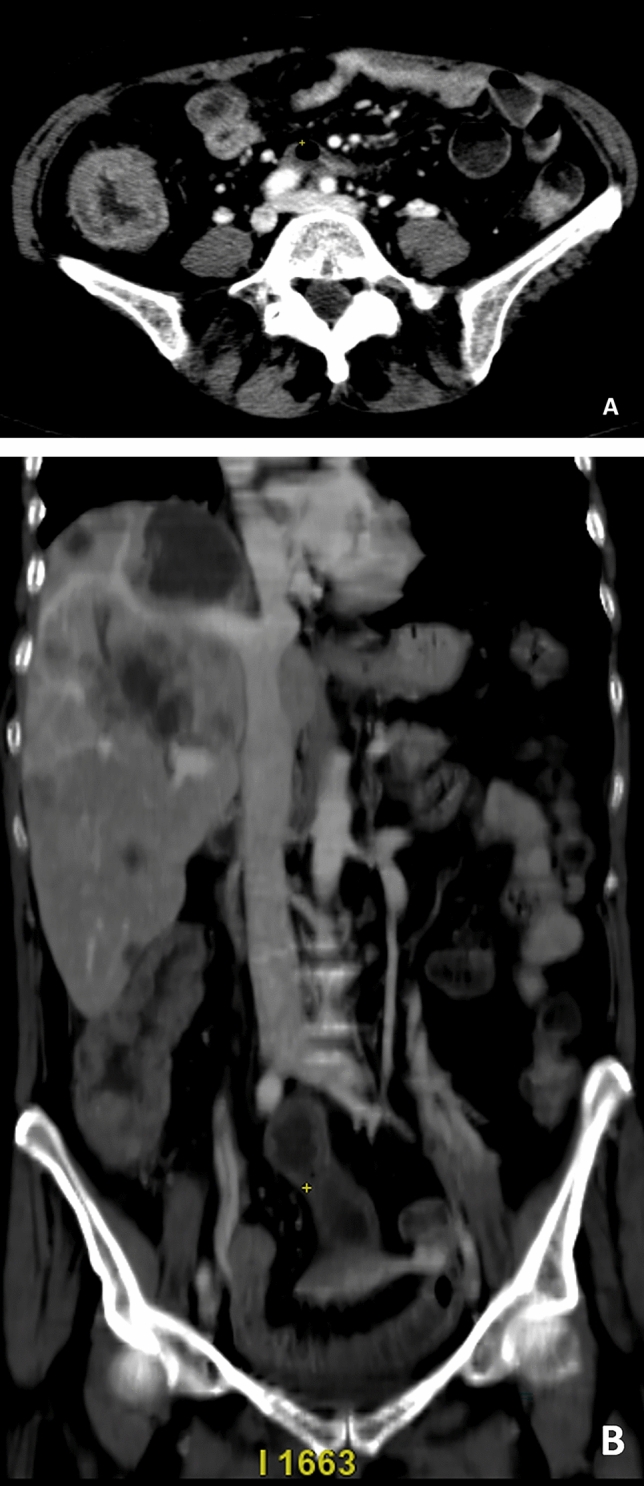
Axial and coronal CT imaging show diffuse wall thickening bowel with or without evidence of the “target sign” and heightened enhancement of the thickened bowel wall: this event is common but nonspecific for bowel ischemia (a and b)
